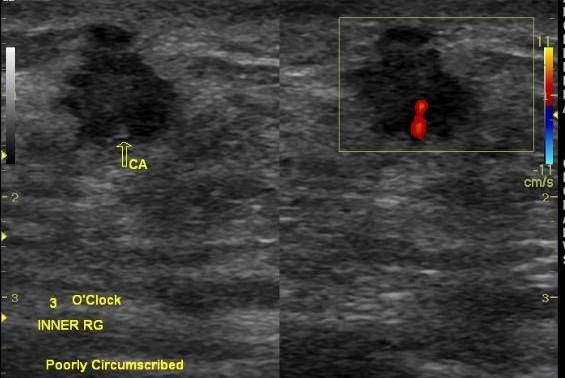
BIRADS 5: (Highly suggestive of Malignancy) Mass on breast Ultrasound
